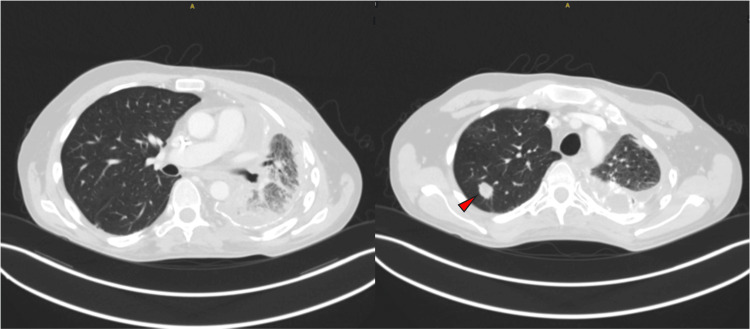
CT chest prior to pazopanib treatment showed clear right lung [A] except for a right upper lobe nodule [B, arrowhead]. Left lung consolidations and pleural scarring are stable from the previous study.
